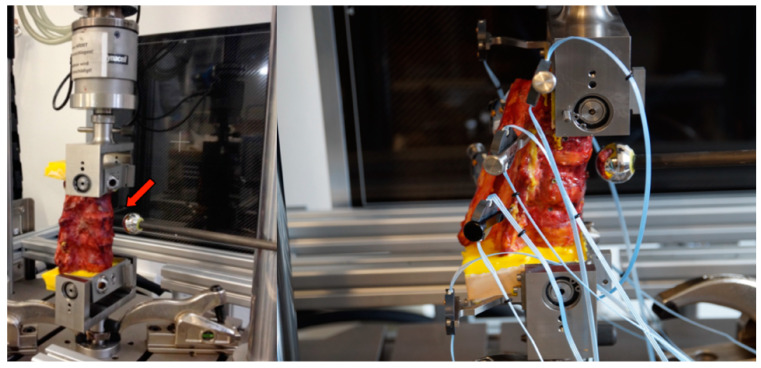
Hydraulic material testing machine used to create standardized incomplete burst fractures and obtain radiographs. At the left, the mounted motion capture marker (rigid bodies) and radiographic reference (arrow) are shown. At the right is a magnified lateral view of a mounted sample.
